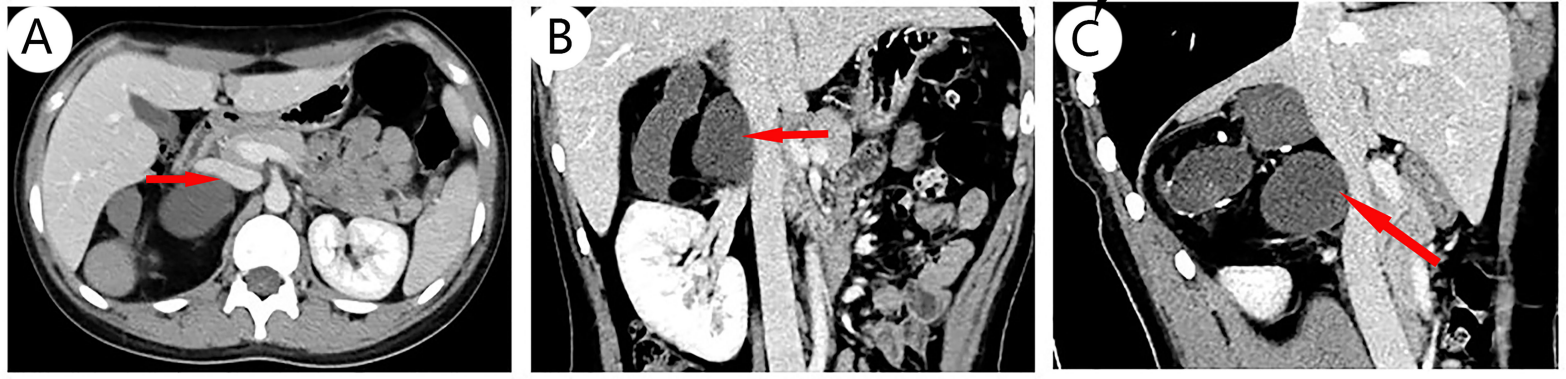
CT scan of blood vessels: No abnormalities in bilateral renal arteries were discovered in CT imaging of renal arteries. A part of inferior vena cava below the lower border of the liver segment is confined by the mass of the right adrenal gland, and the venous lumen is a local stenosis; different layers show that the vena cava is compressed, including transverse [(A), arrow], coronal [(B), arrow], and sagittal planes [(C), arrow].
